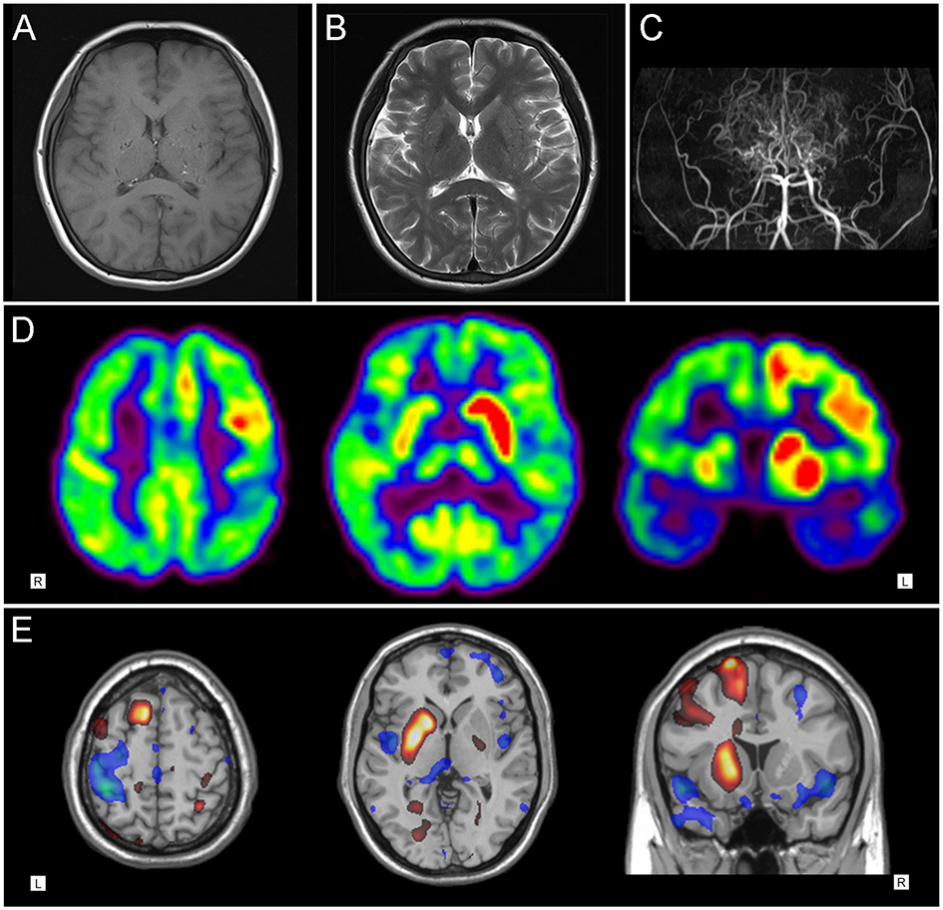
Neuroimaging findings in patient 1. (A) T1-weighted and (B) T2-weighted axial cranial magnetic resonance imaging showed multiple flow-voids on both basal ganglia. (C) Magnetic resonance cerebral angiography revealed severe stenosis in the proximal circle of Willis, with numerous collateral vessels. (D)
